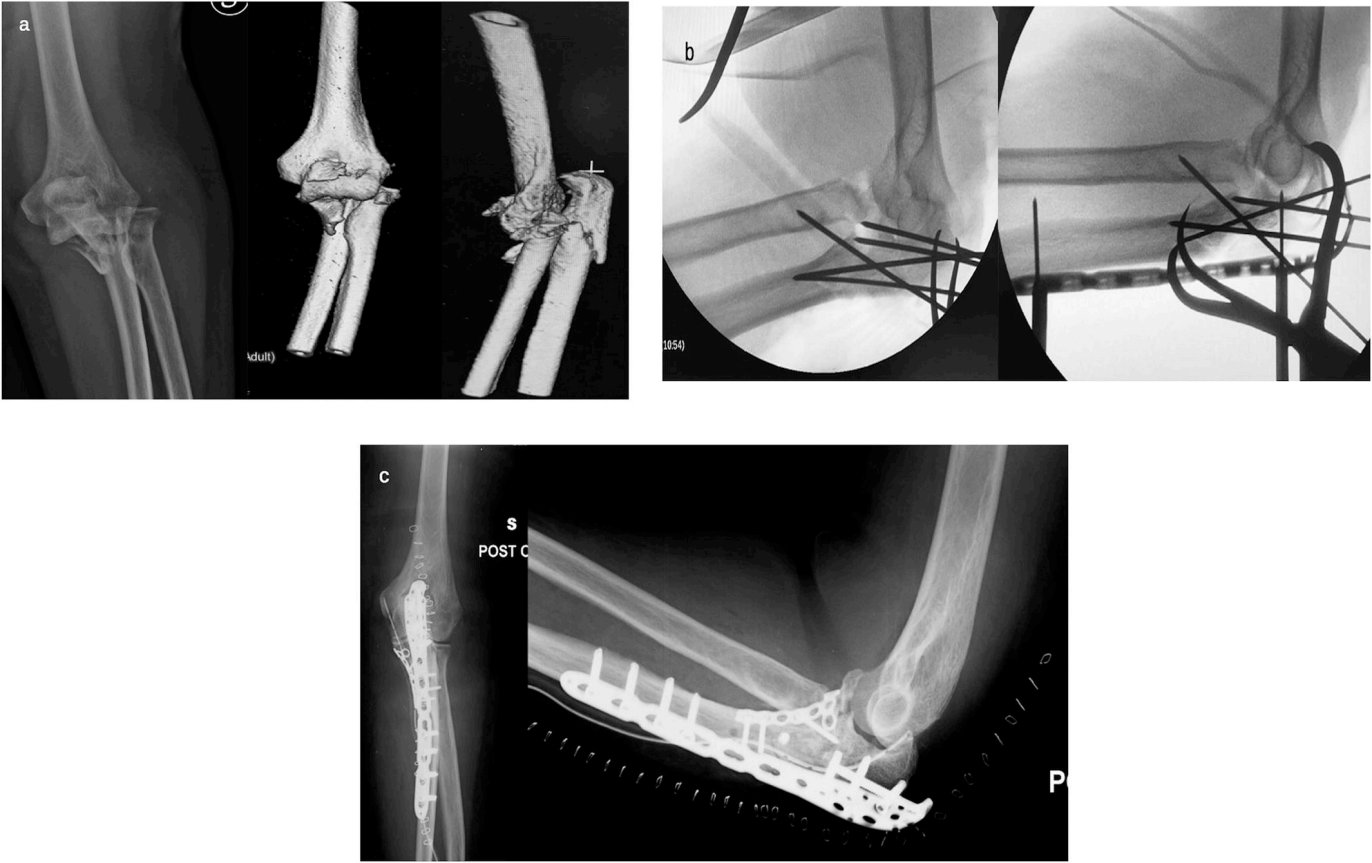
a) Radiographs and 3D-CT scan: fracture-dislocation of ulno-humeral joint, comminuted fracture of proximal ulna and sigmoid notch; large fragment of coronoid process. Fracture of the radial head with a small fragment. Osteoporosis. The patient was an 86-year-old woman. b) Intraoperative fluoroscopy: Taylor-Scham approach - temporary fixation of the fragments with clamps and K-wires. c) Post-operative radiography: Pre-contoured locking compression plate and 2.4 T buttress plate for coronoid fracture. Removal of the small fragment of the radial head.
